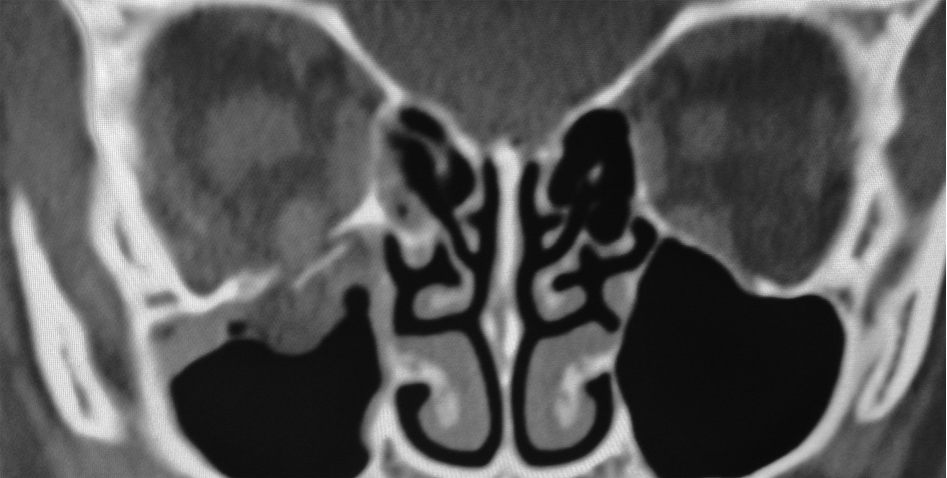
CT showing fracture of the orbital floor with muscle herniating into the defect.
